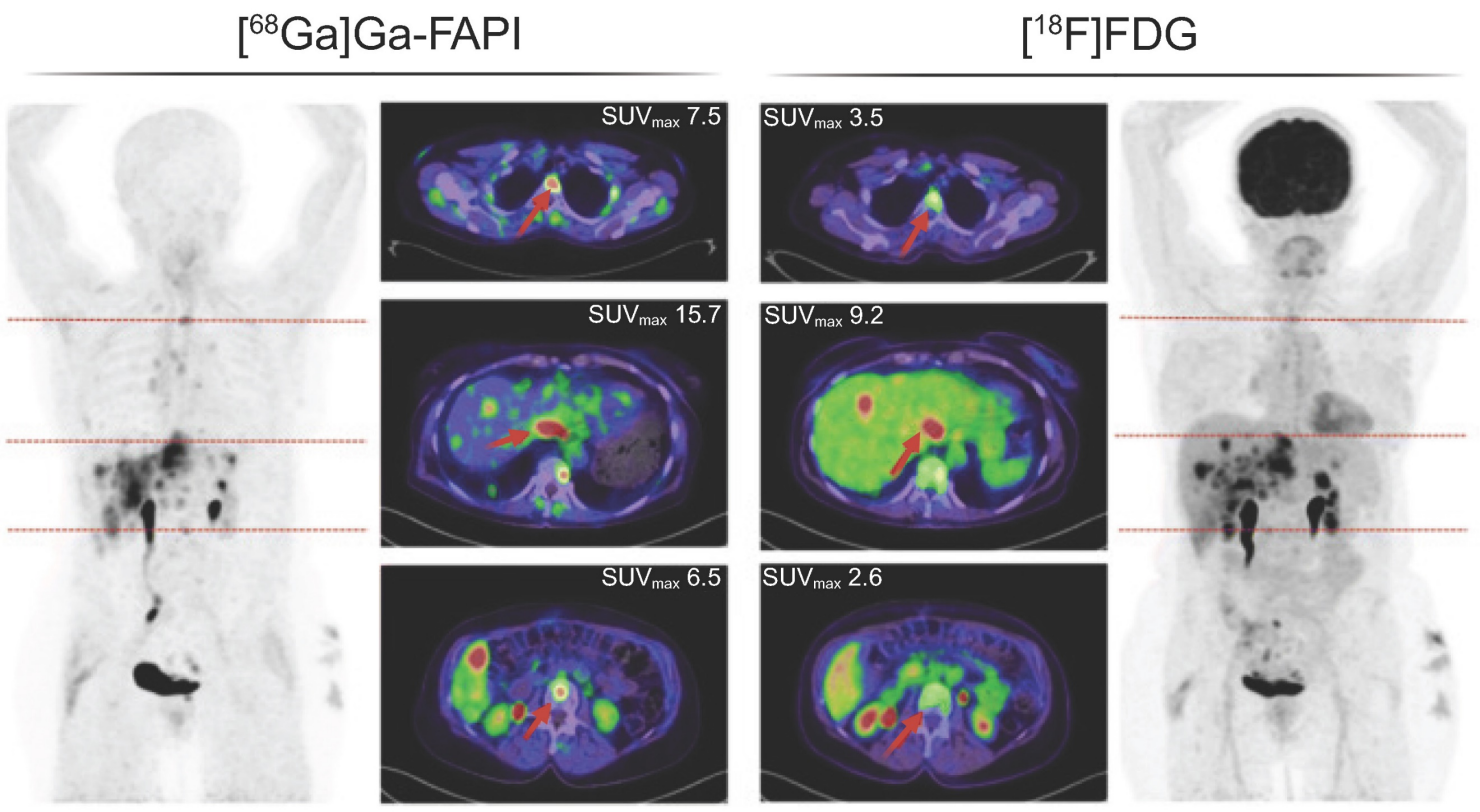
[^68^Ga]Ga-FAPI-PET/CT followed by a [^18^F]FDG-PET/CT one month later in a 63-year-old woman with metastasized ovarian cancer. The normal brain parenchyma and liver depict a clear visual difference in tracer uptake. Adapted with permission from Dendl *et al*. 21 under the Creative Commons Attribution 4.0 International License, © Springer Nature. FAPI: fibroblast activation protein inhibitor; [^18^F]FDG: [^18^F]fluorodeoxyglucose; SUV_max_: maximal standard uptake value.
